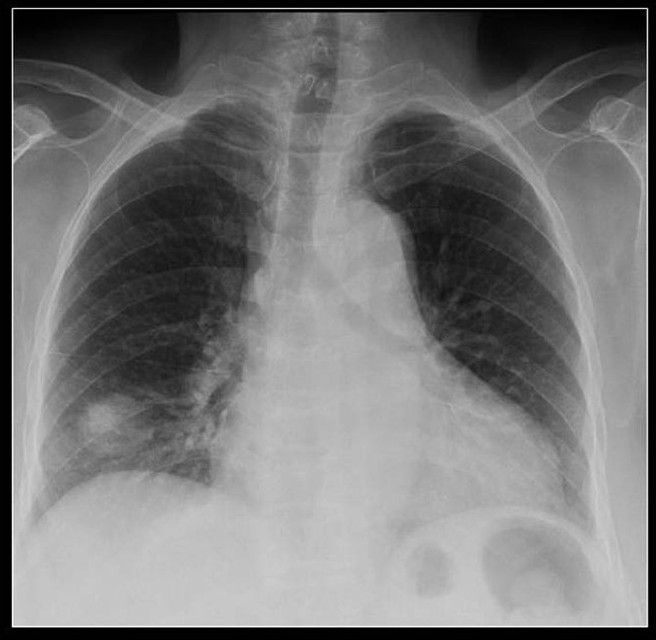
A chest radiograph showing a large PN at the right lung base with central high density due to calcification in a metastatic deposit from a leiomyosarcoma of the uterus
